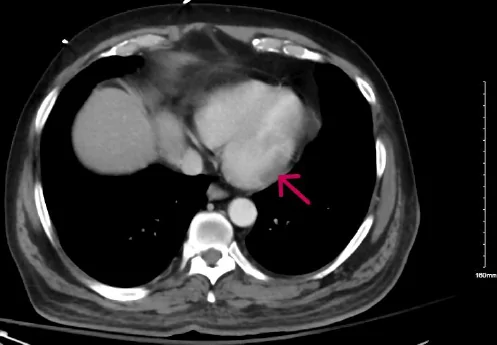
Computed tomography of chest with intravenous contrast showing findings consistent with possible ischemia versus motion artifact to the left heart. The red arrow points to the area of hypoperfusion located in the left lateral ventricle base.
